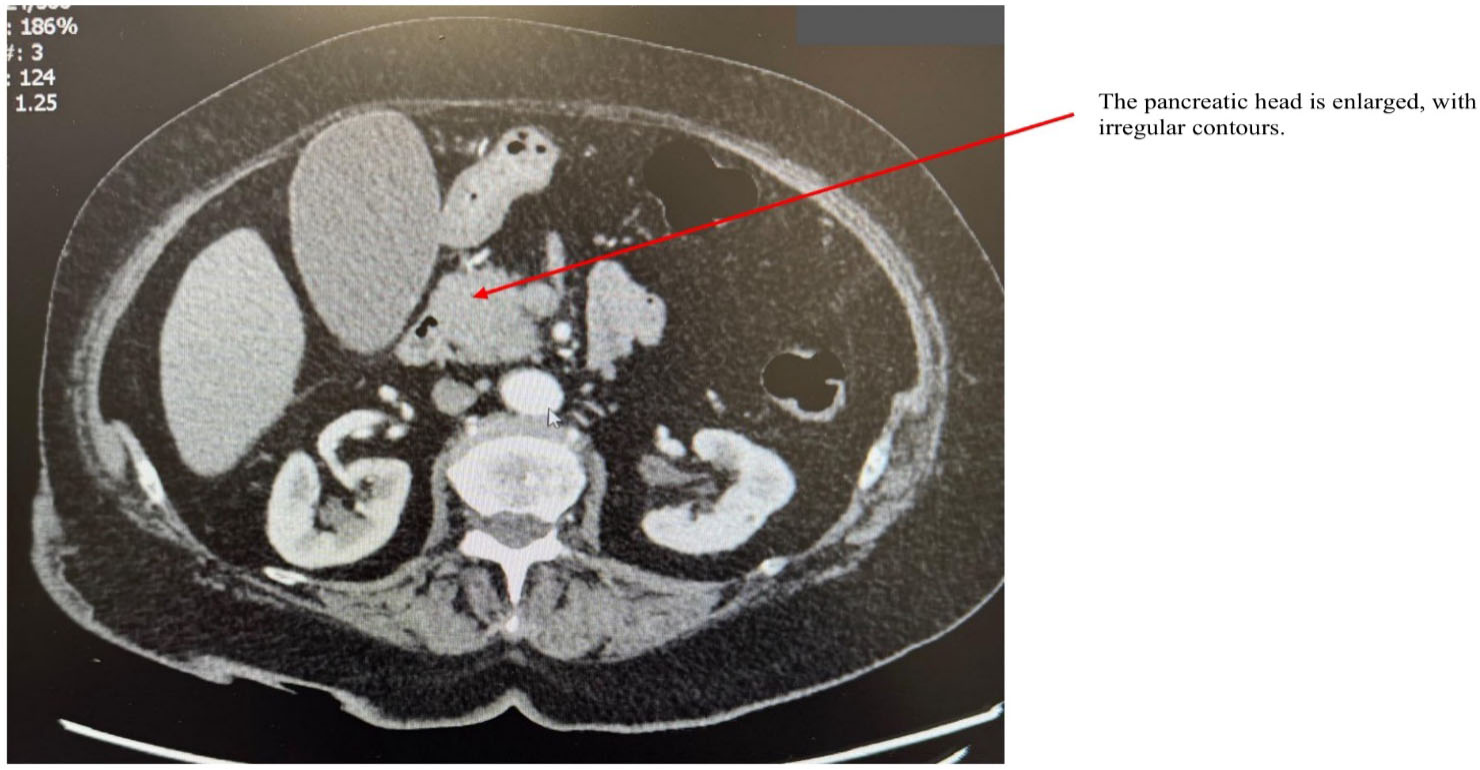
CT scan of a patient with AIP. The arrow points to an enlarged pancreatic head with irregular contours.
